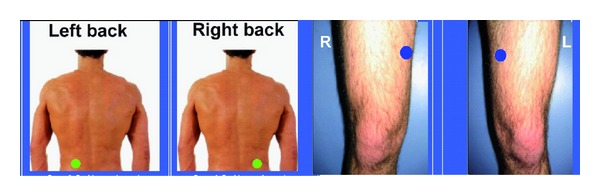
Ultrasound measurement points at the posterior abdominal wall and midthigh (right and left).
